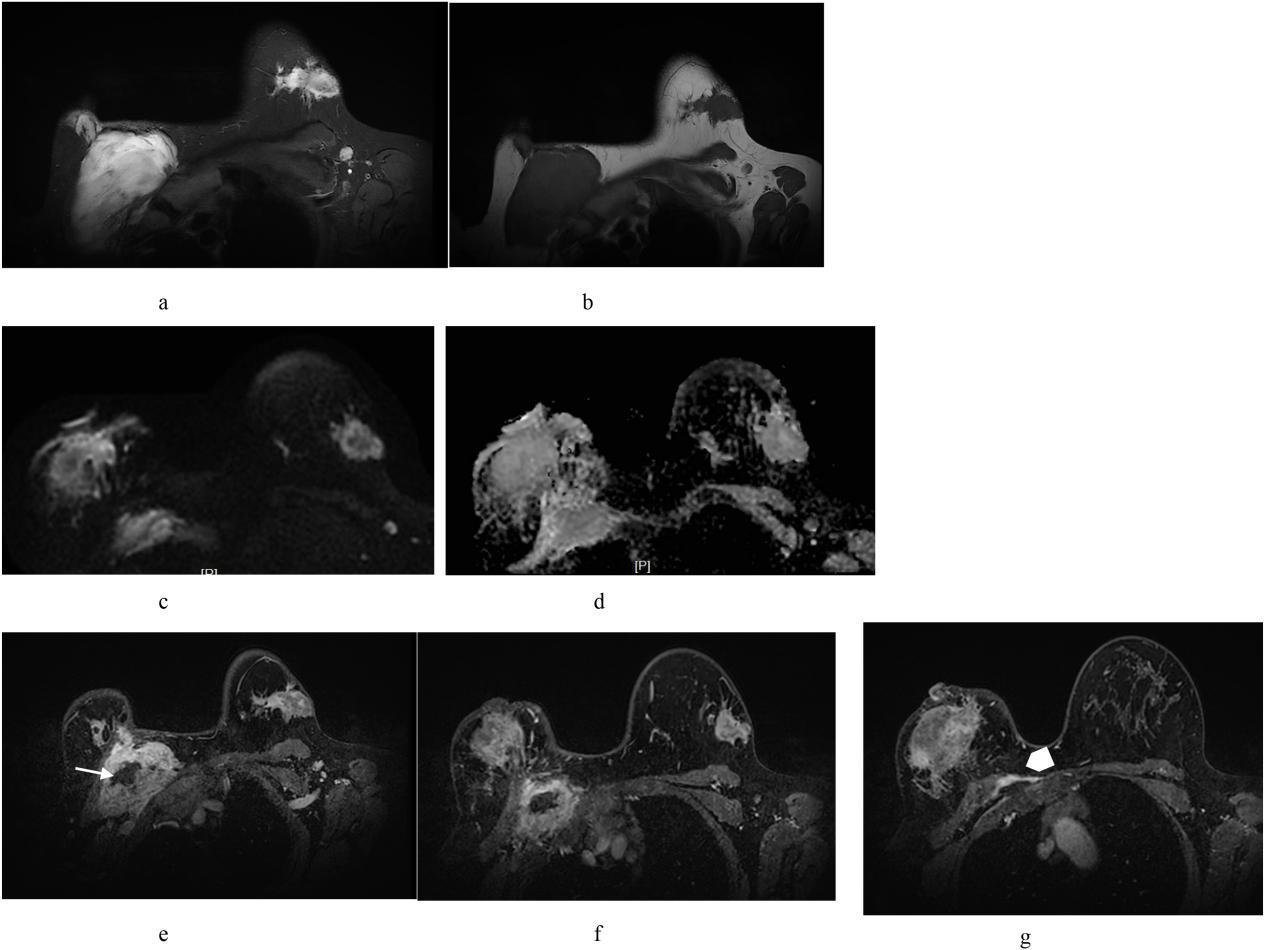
Contrast-enhanced breast magnetic resonance imaging findings. Bilateral breast masses: Irregular lesions with spiculated margins were observed (a, b). Diffusion-weighted imaging (DWI) (c) demonstrated restricted diffusion with reduced apparent diffusion coefficient values (d). Post-contrast sequences revealed heterogeneous enhancement (e-g). Right breast mass: Ill-defined borders and chest wall adhesions were observed. A large, irregularly shaped mass with a heterogeneous signal intensity was observed between the right pectoralis major and minor muscles. This lesion exhibited DWI characteristics and enhancement kinetics similar to those of primary breast masses, along with a “band sign” (linear hypointense bands) [(e) white arrow] and “fascial tail sign” (fascial extension) [(f) white arrowhead] at the interface with adjacent musculature.
